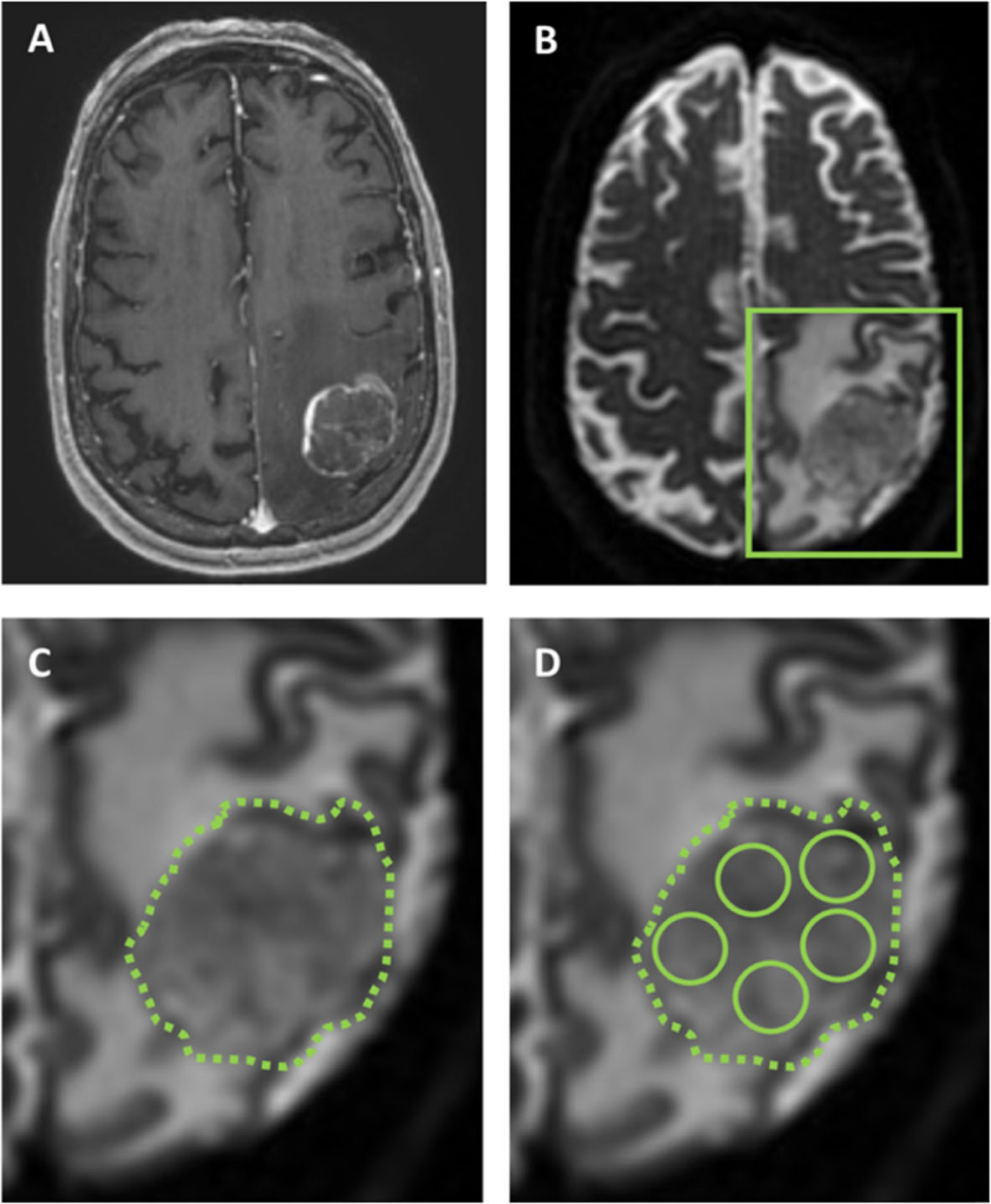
Example of measurement of tumor ADC by manual placement of regions of interest A patient with a history of lung adenocarcinoma presents with headache and focal neurological deficit. a. T1 weighted MRI with gadolinium demonstrates a left parietal lesion, which was confirmed as a metastasis by pathology. b. ADC map is generated from DWI using post processing software and fused to the T1 post contrast study. C and D are zoomed images of B as outlined in green. c. A region of interest is traced around the tumor border using the T1-weighted post gadolinium scan and then applied to the ADC map. d. Regions of interest of an agreed size - here five circles of 50mm^2^ -are placed within the tumor on the axial slice with the largest area, avoiding necrosis, haemorrhage or cyst, and the overall mean is calculated
